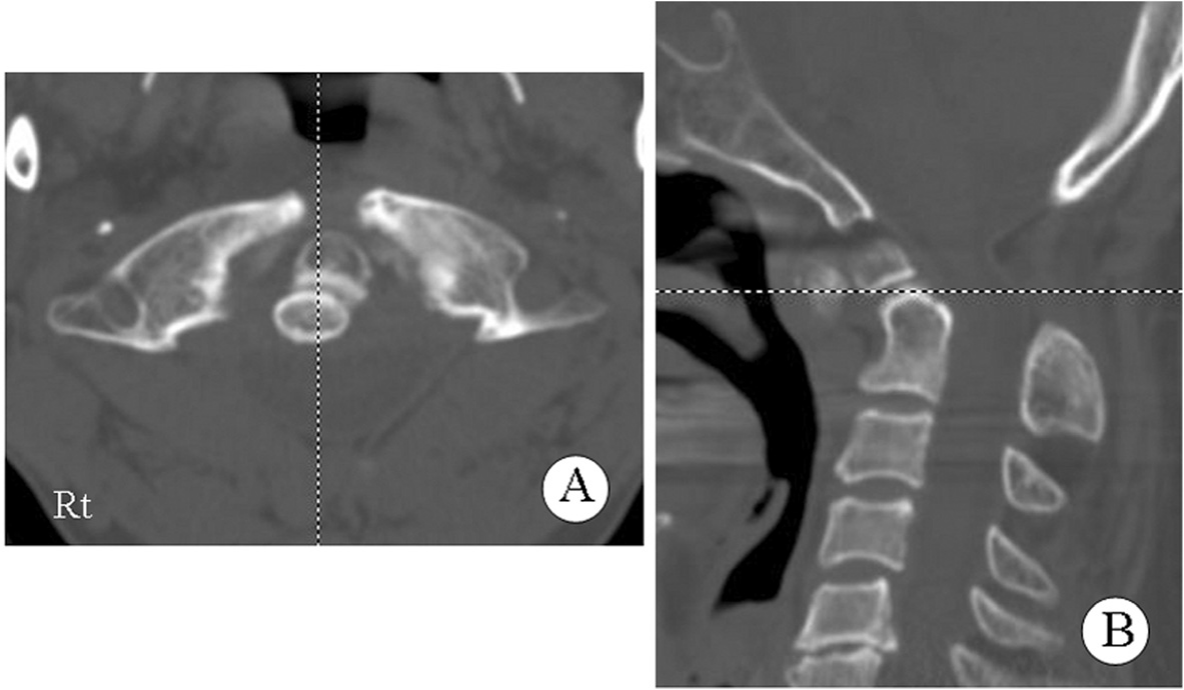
Computed tomography images of the cervical spine. (A) Complete absence of the posterior arch of the atlas with an anterior cleft was observed. (B) The sagittal plane of the three-dimensional computed tomography scan shows the os odontoideum.
